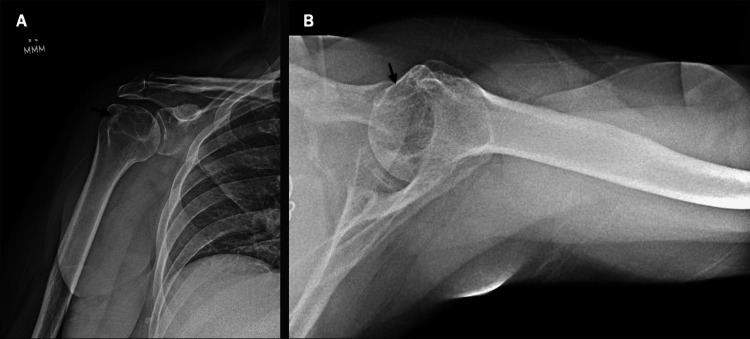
Anteroposterior (A) and axillary lateral (B) radiographs with black arrows are shown in panels A and B, demarcating the large lytic lesion at the superior aspect of the right humeral head adjacent to the greater tuberosity.
